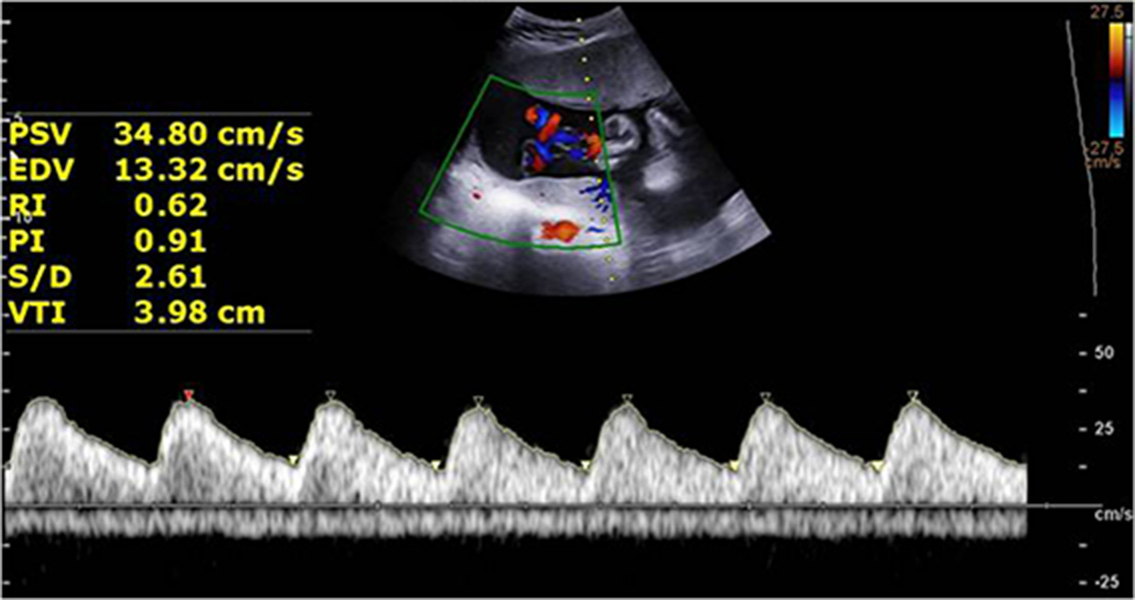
Doppler measurement of the UA. Abbreviations are as in Figure 4.
